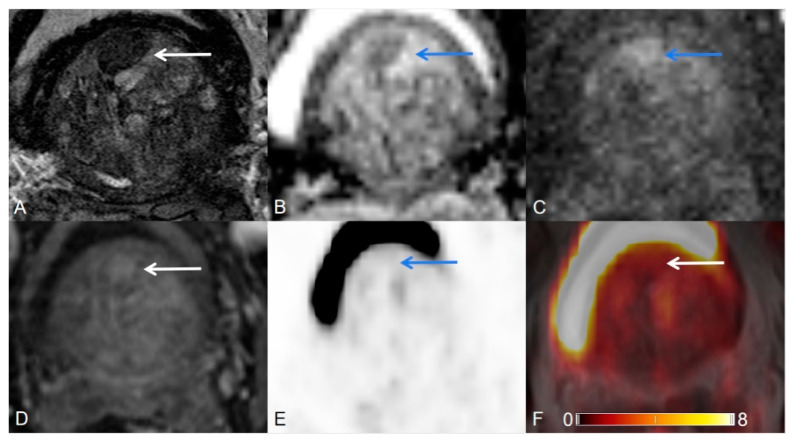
Transition zone with an atypical nodule. (A) Axial T2WI shows a homogeneous T2 hypointense, mostly encapsulated nodule. (B) ADC map image presents a focal lesion with a markedly hypointense signal below the background corresponding to the lesion seen in (A). (C) DWI (b = 1000 s/mm^2^) shows a focal lesion with a markedly hyperintense signal above the background corresponding to the lesion seen in (A,B). (D) Early dynamic contrast-enhanced image presents avid enhancement within the nodule. T2WI = 2, DWI = 5, DCE = positive, PI-RADS assessment category = 3. (E) PET image shows no [^68^Ga]Ga-PSMA-11 avid uptake. (F) [^68^Ga]Ga-PSMA-11 PET/MRI fusion.
